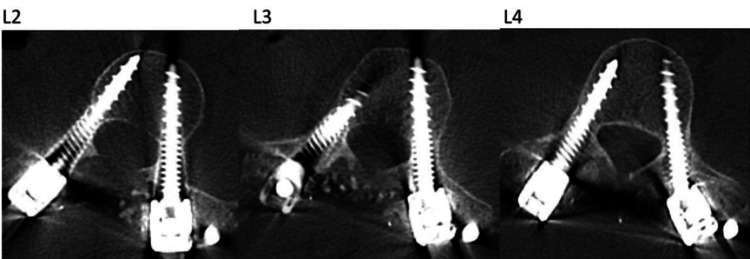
Postoperative CT imaging demonstrating accurate pedicle screw instrumentation at the L2 through L4 apical levels.
